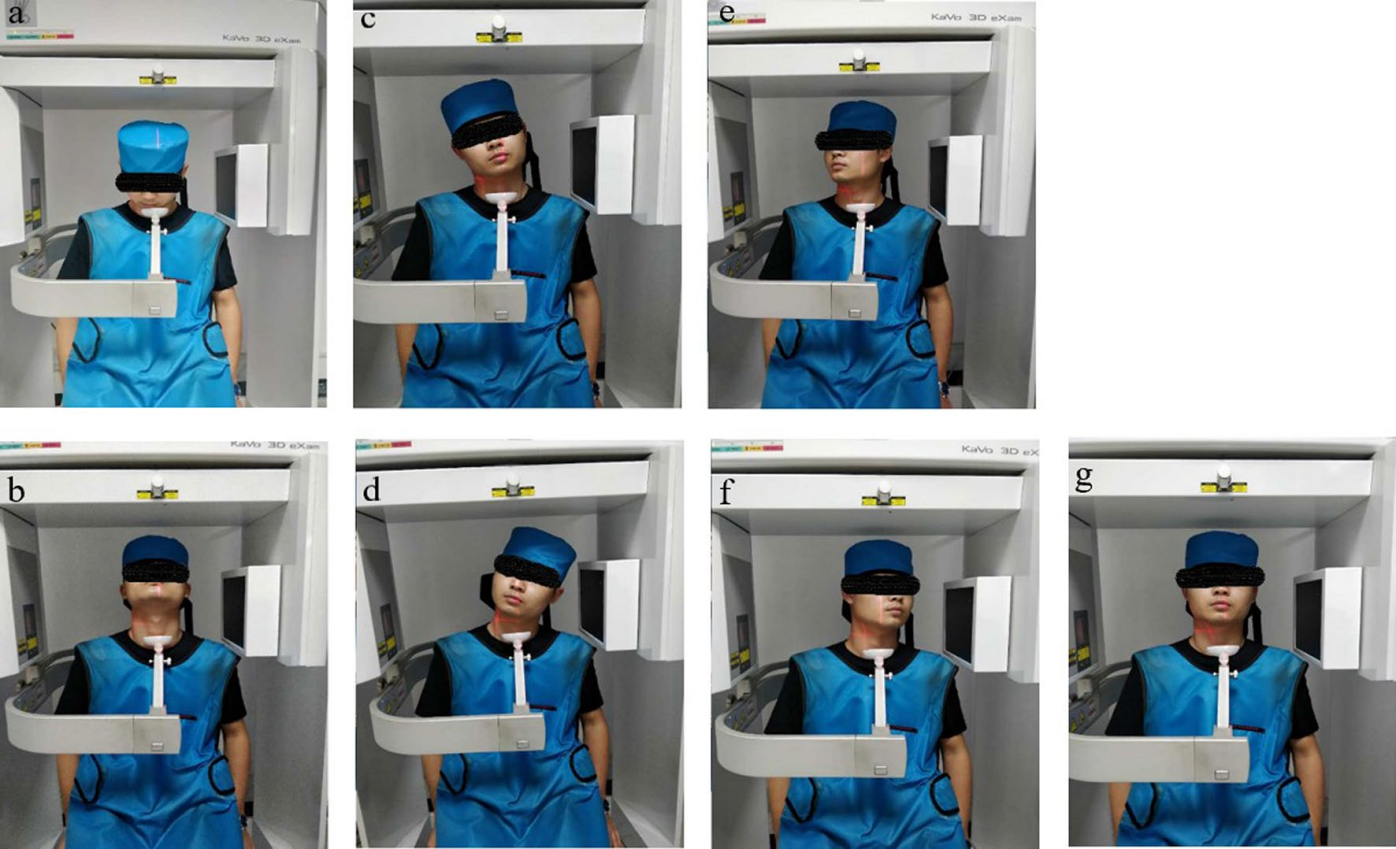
Overview of cone-beam computed tomography (CBCT) scanning showing a participant performing (a,b) flexion–extension (F–E), (c,d) lateral bending (LB), and (e,f) axial rotation (AR) movements, as well as (g) a neutral position.
